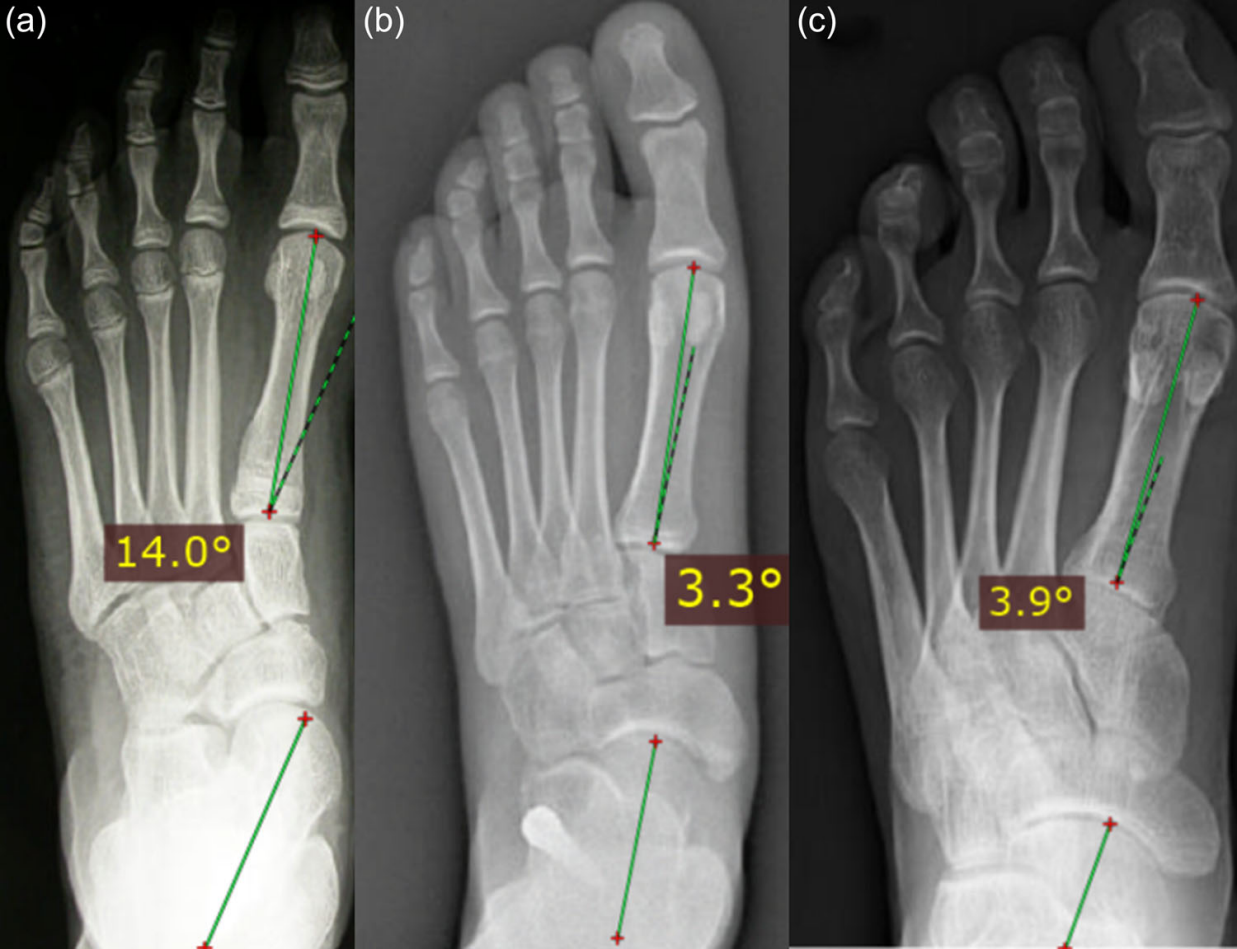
Talar–first metatarsal angle, between the lines drawn along the long axis of the talus and the first metatarsal in antero‐posterior radiographic view; before correction (a), with the screw implanted (b) and 11 years after screw removal (c).
